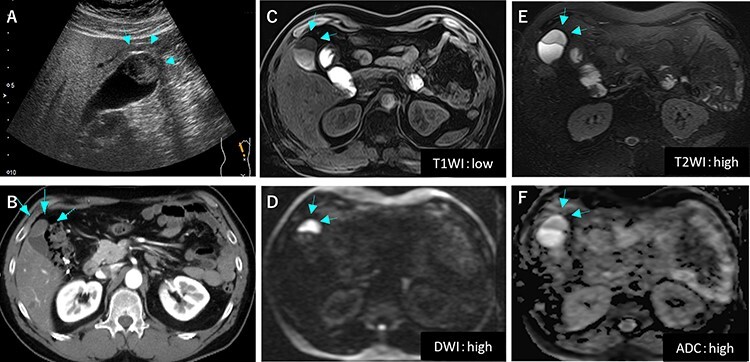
Preoperative image findings; (A) Gallbladder tumor was at gallbladder body by abdominal ultrasonography; (B) CT scan revealed slightly enhanced tumor located at gallbladder fundus; (C–F) On MRI, the tumor had high signal in both of T2 and diffusion weighed image and low signal in both of T1 and apparent diffusion coefficient.
